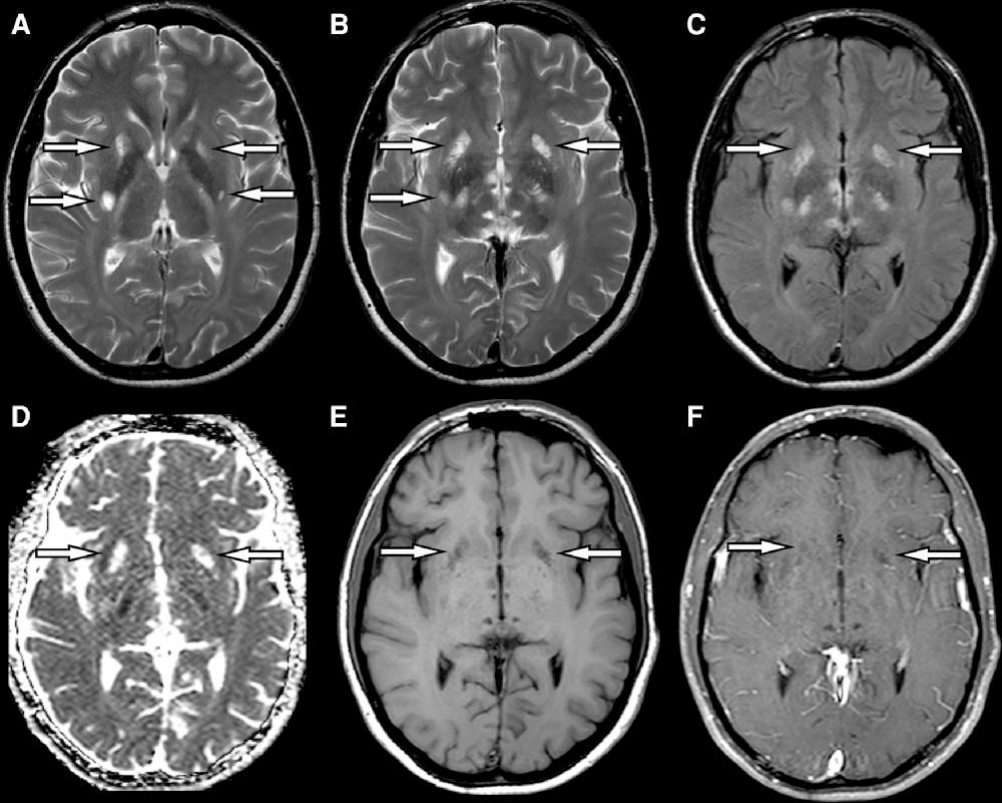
Brain MR from patient 10 (A–F), demonstrating putaminal involvement. Axial T2-weighted images (A, B) demonstrate hyperintense foci in the ventral and dorsal portions of both putamina (arrows). Axial FLAIR image (C) discloses the same hyperintensity (arrows). These foci present facilitated diffusion, characterized by hyperintensity in the corresponding ADC map (D, arrows). Axial T1 (E) and post-contrast T1 (F) demonstrate these foci as hypointense, without enhancement (arrows).
